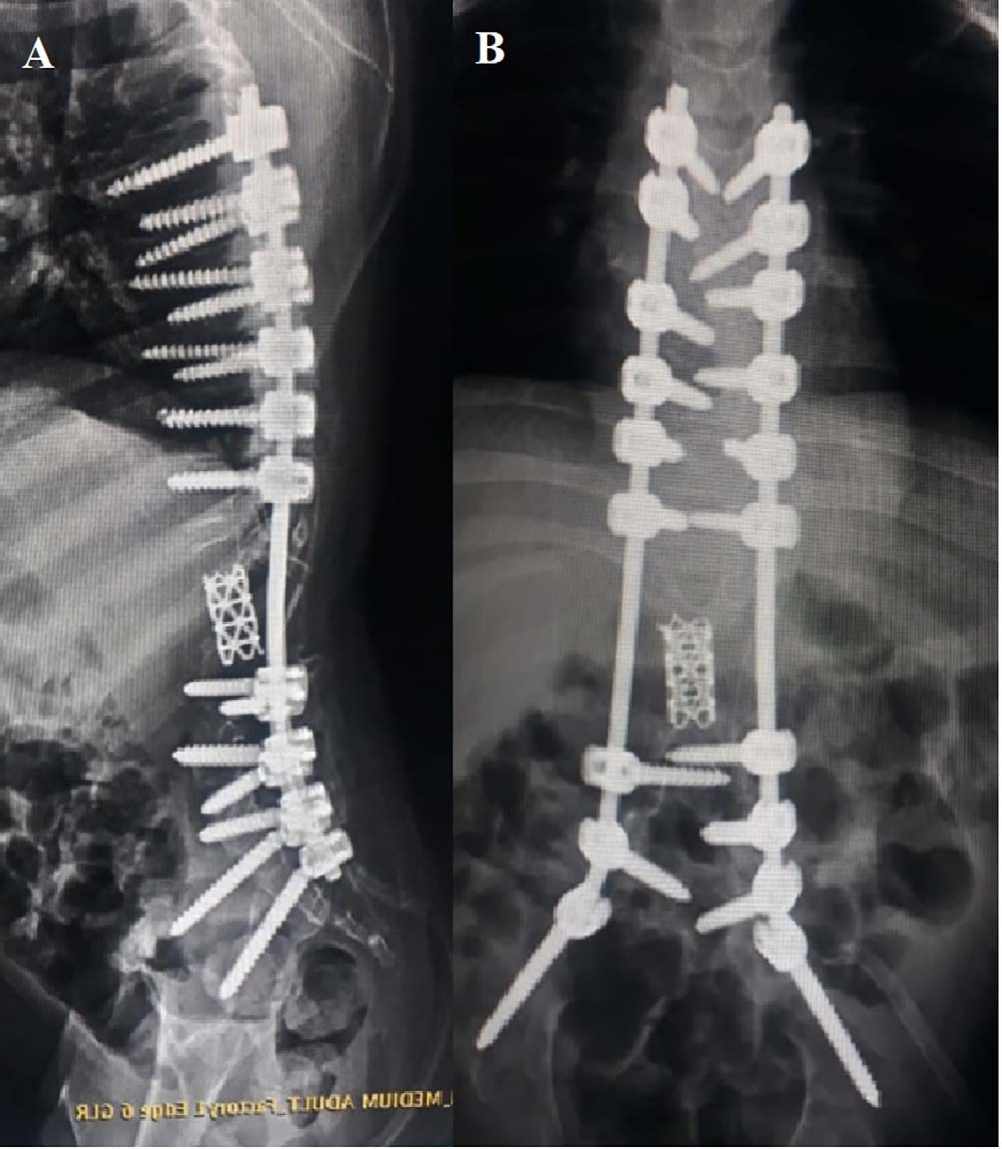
Postoperative. (A) Lateral plain X-ray showing kyphectomy/titanium mesh cage inserted. (B) Anteroposterior plain X-ray showing S2 alar iliac screws/titanium cage.
